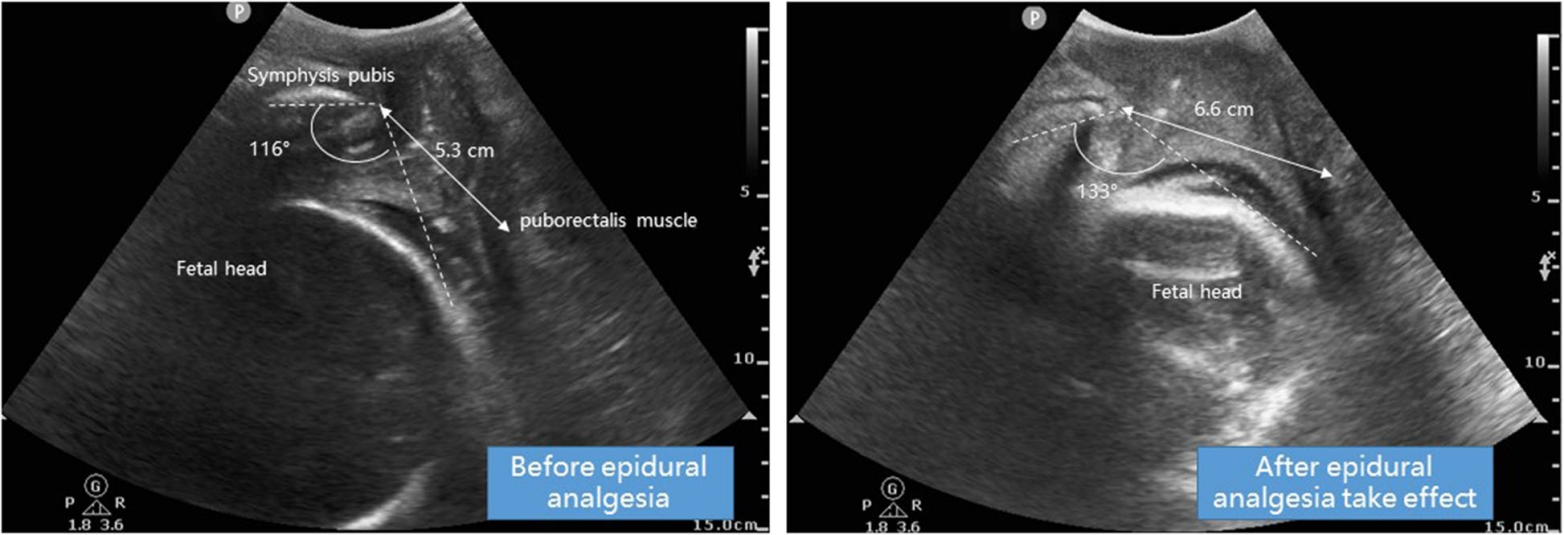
The variation of transperineal ultrasound measured at rest with fetal heart deceleration associated with initiation of epidural labor analgesia. Transperineal ultrasound (TPU) was performed before epidural analgesia and after epidural analgesia was attained. The anteroposterior diameter of the levator hiatus (APD) increased from 5.3 to 6.6 cm and angle of progress (AoP) increased from 113° to 133°
